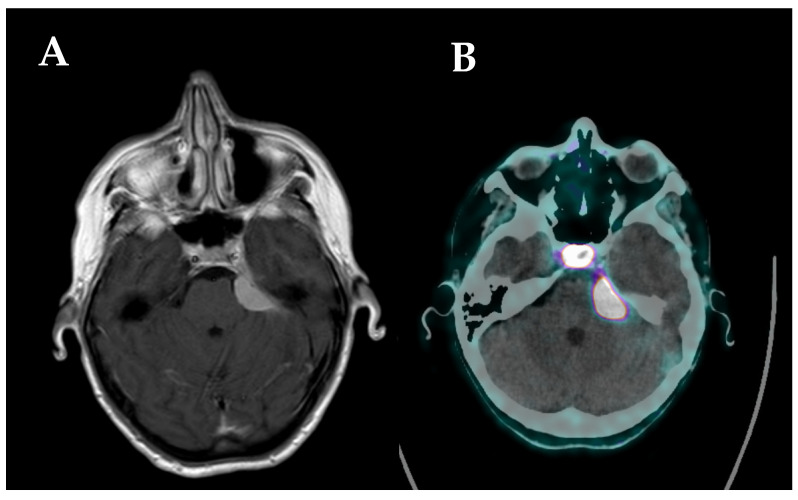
(A) MRI scan showing a left sphenopetrosal lesion suspected to be meningioma; (B) [^68^Ga]Ga-DOTATOC PET/CT scan showing high tracer uptake of the left sphenopetrosal lesion, suggesting the diagnosis of meningioma, normal tracer uptake of the pituitary gland.
